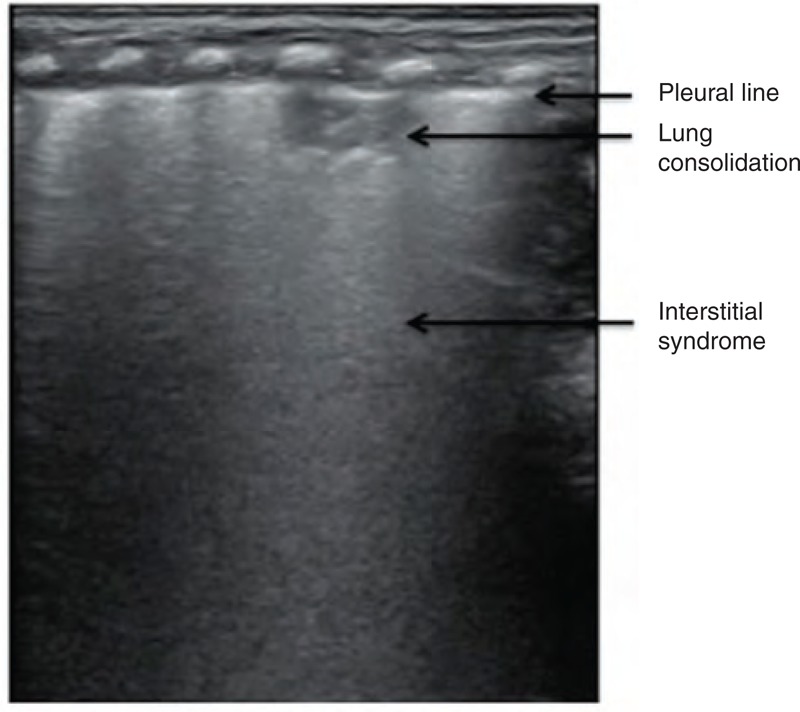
Lung edema with consolidation. An ultrasound showed a small area of focal consolidation beneath the lung pleura (possibly atelectasis or inflammation), whereas the remaining lung field demonstrated interstitial syndrome, suggesting the presence of mild lung edema.
